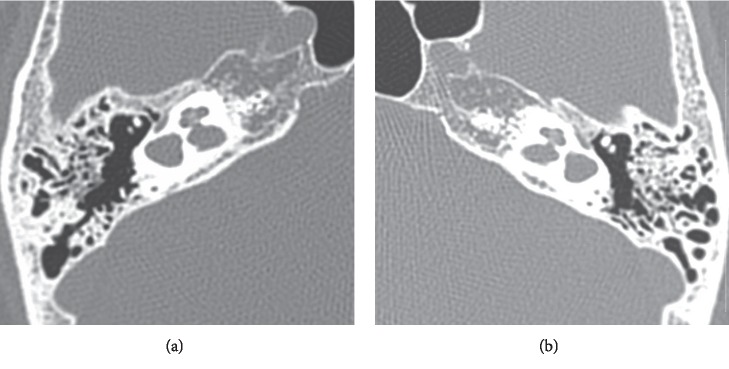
HRCT. The fusion of the vestibules and the lateral semicircular canals. (a) Right ear. (b) Left ear.
